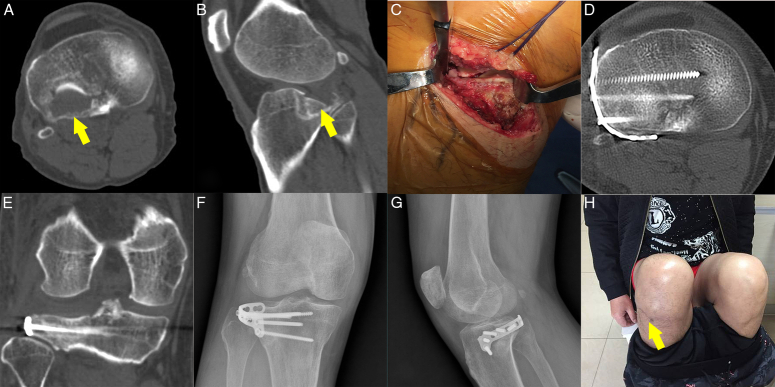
The therapeutic protocol for PLF cases. (A,B) Preoperative CT images demonstrating collapse in the posterolateral quadrant. (C) Adequate visualization of the PLF was achieved through the supra-fibular head approach. (D,E) Immediate postoperative CT images showing the collapsed articular surface effectively elevated using a horizontal belt rafting plate. (F,G) Postoperative X-rays confirm good anatomical reduction. (H) Satisfactory functional restoration was observed at the final follow-up, 12 months postoperatively. PLF, posterolateral tibial plateau fracture.
